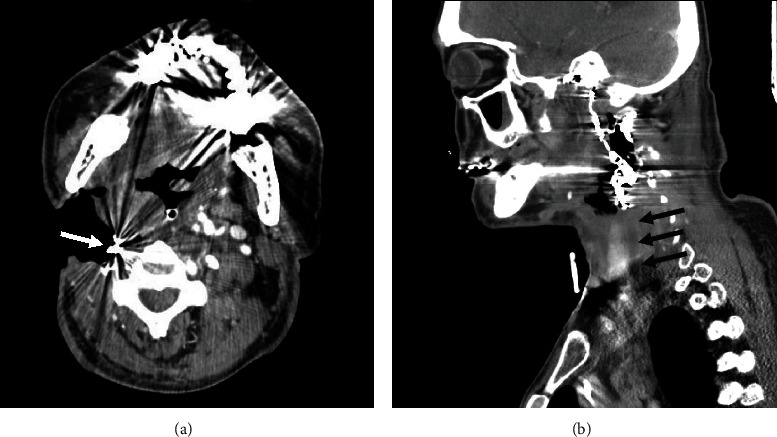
Computed tomography angiography showed (a) exposed embolization coils (white arrows) in the malignant wound of right neck and (b) thrombosed right common carotid artery (black arrows).
